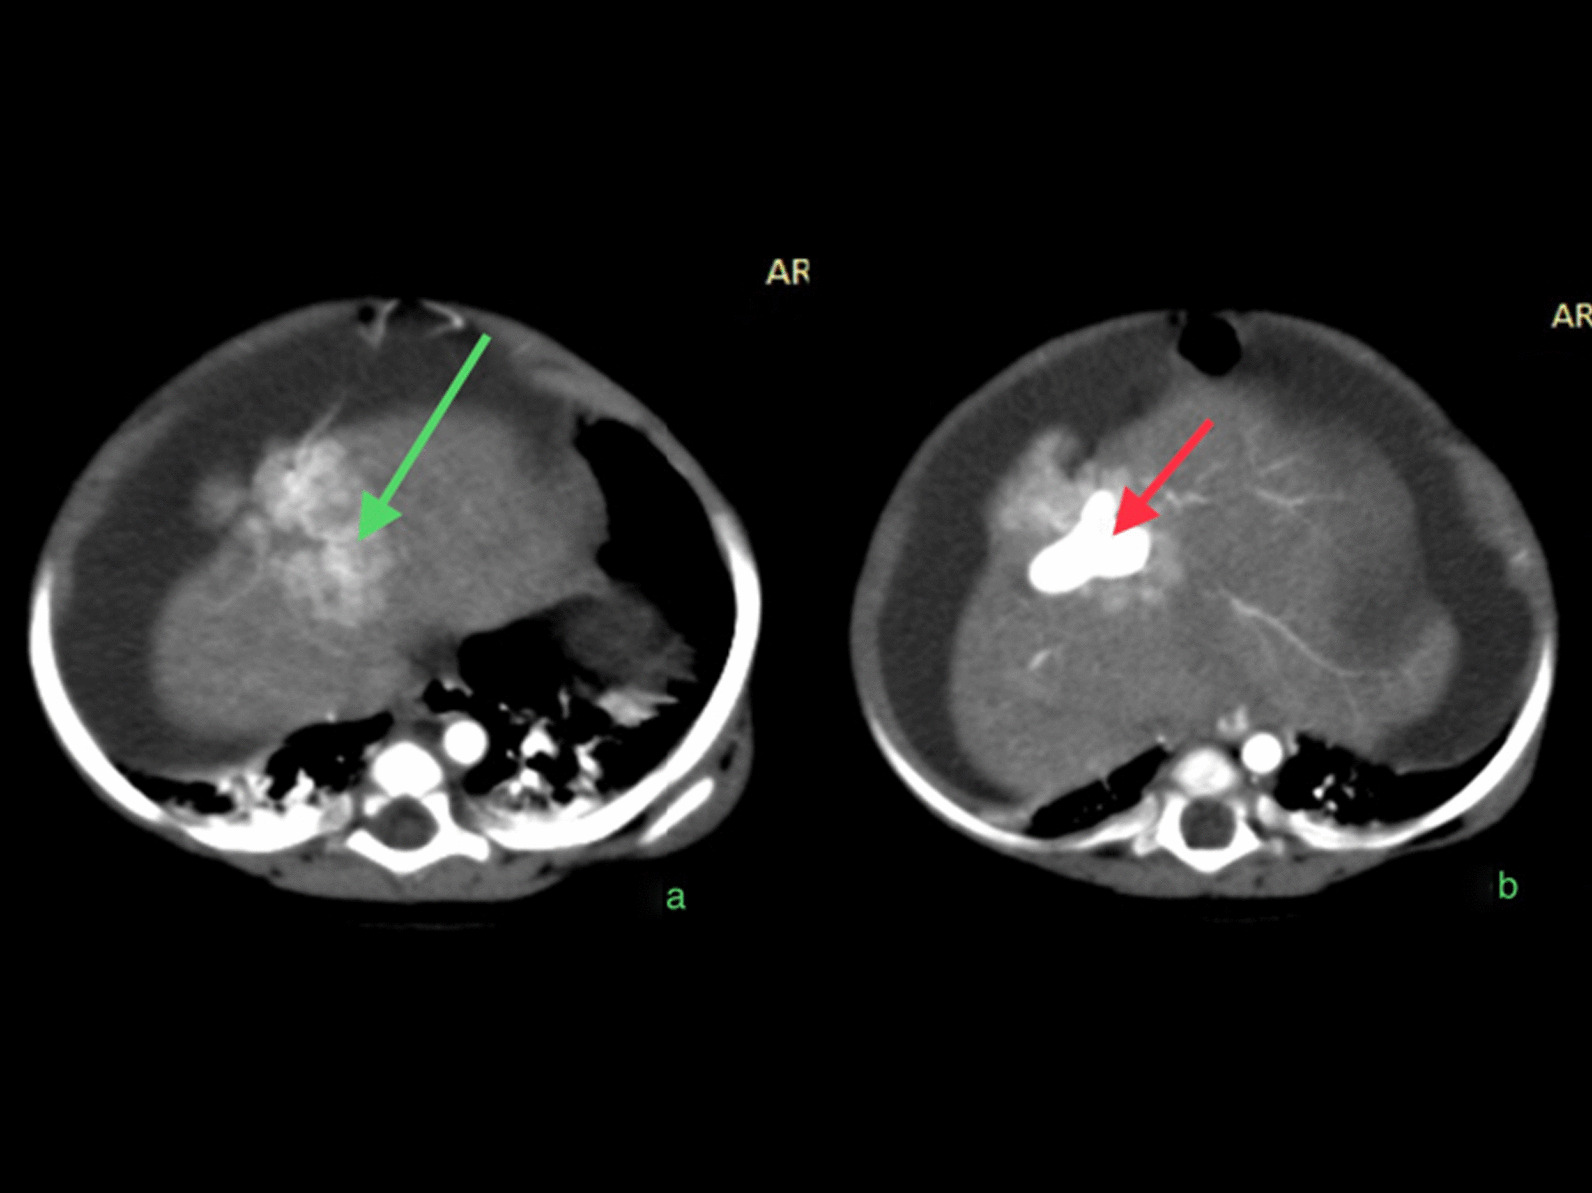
Multislice CT hepatic angiography and portography; a soft tissue enhancing mass (Green arrow), b intensely enhancing cystic lesion (vascular space) enhancing during the arterial phase vascular arterial lesion (red arrow)
